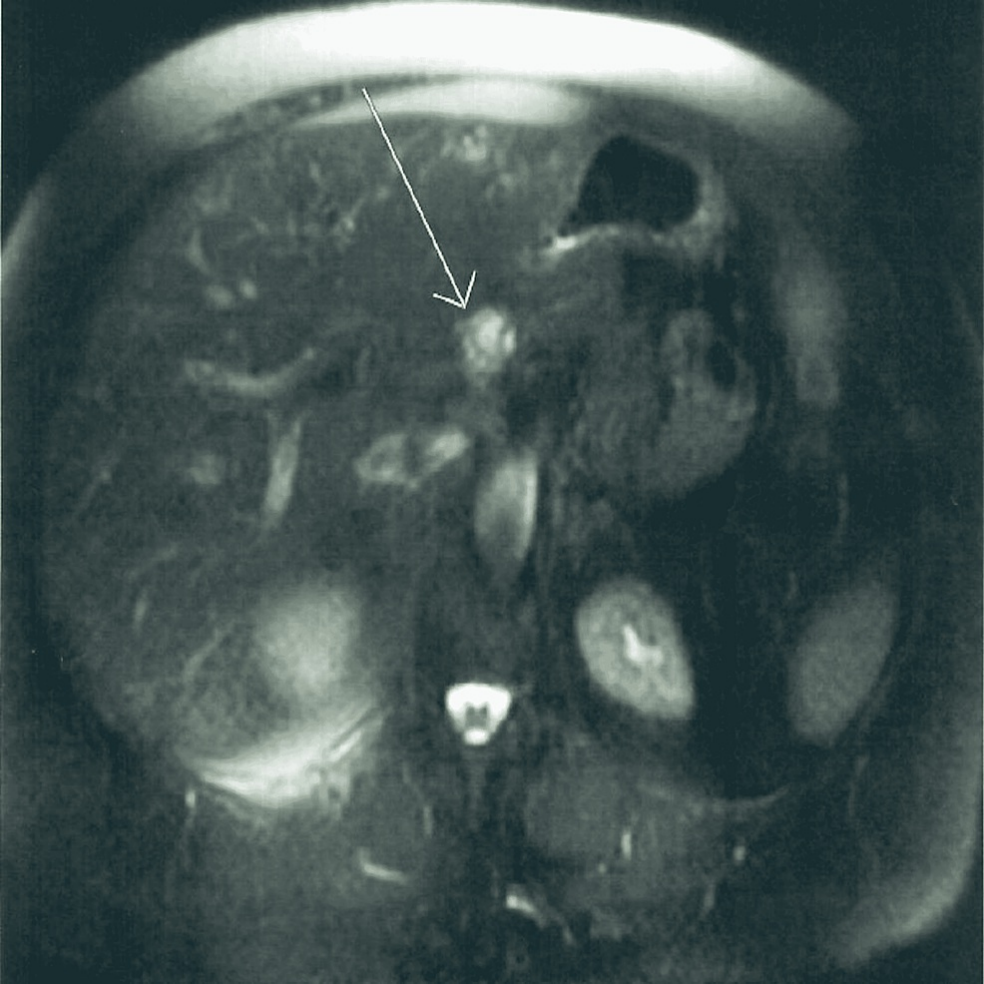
Pancreatic schwannoma on magnetic resonance imaging of the abdomen (arrow).
